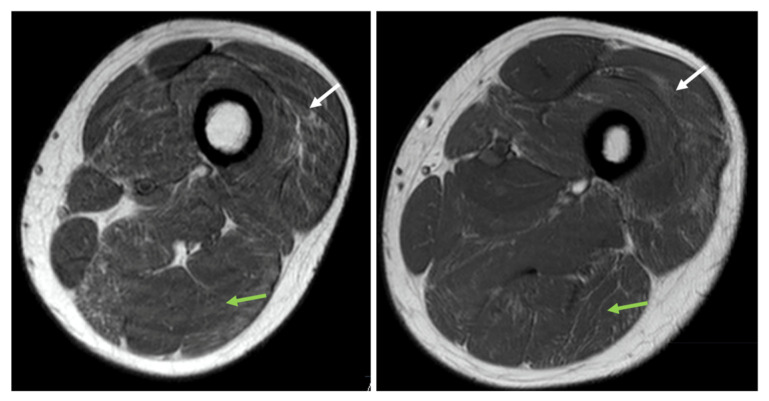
MRI T1 axial view of the left upper leg at two levels. There is fatty infiltration of the vastus lateralis (white arrow) and biceps femoris (green arrow), most pronounced in the vastus lateralis and biceps femoris compartments of these muscles.
